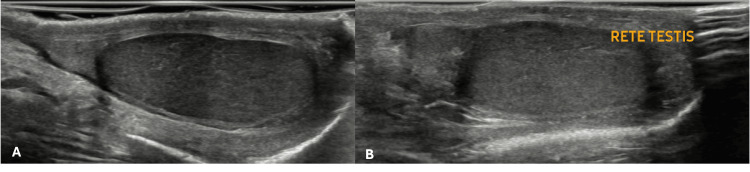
Scrotal ultrasound in a paediatric patient with biochemical evidence of CAH demonstrating normal testicular morphology, consistent with preclinical (Stage I) disease. (A) Right testis showing normal size and homogeneous echotexture without focal intratesticular lesions. (B) Left testis showing normal size and echotexture without focal intratesticular lesions. CAH: congenital adrenal hyperplasia
